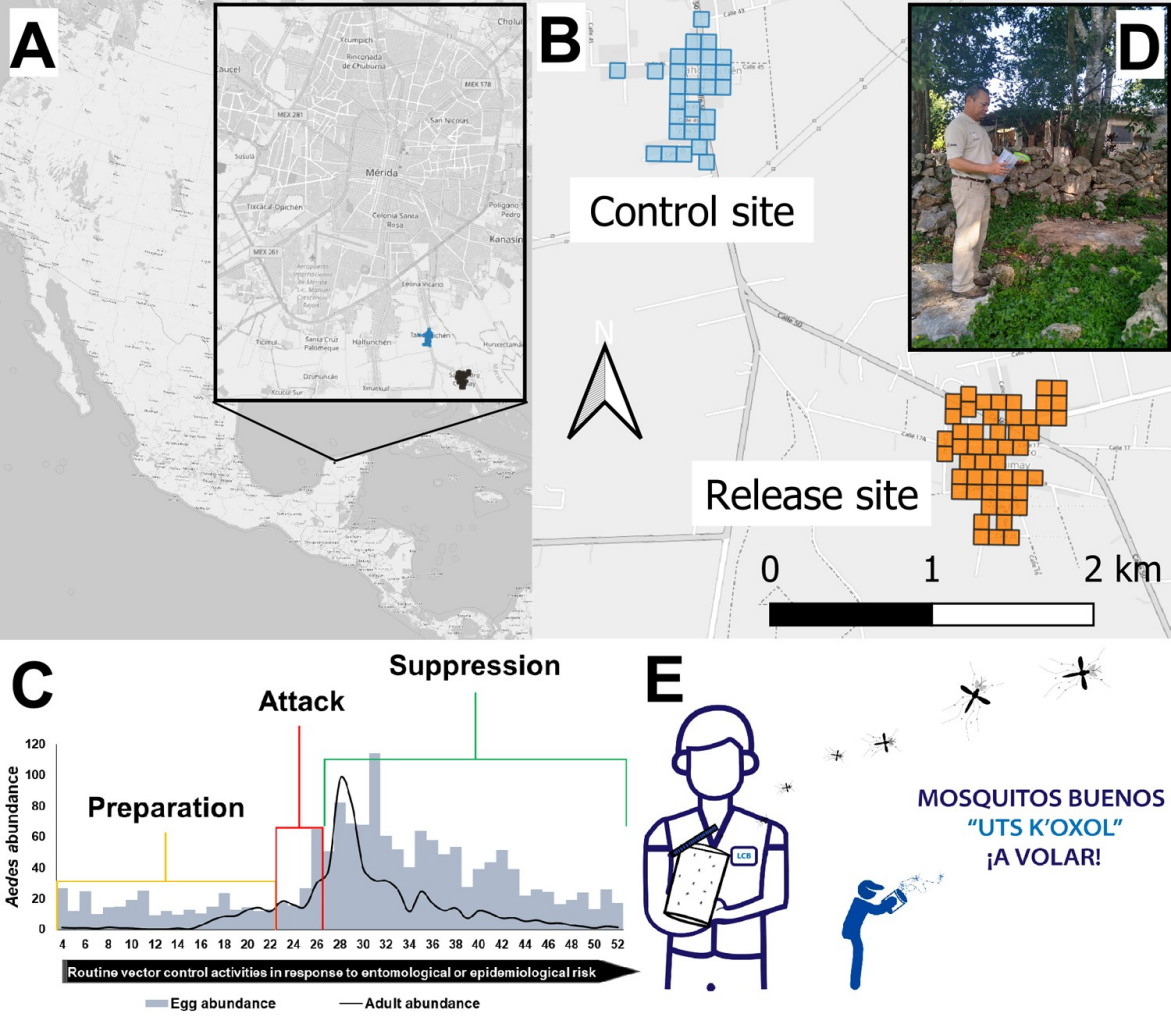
A) Field sites located in the periphery of the city of Merida, southeast of Mexico; San Pedro Chimay (release site) shown in orange and Tahdzibichén (control site) in blue; B) Distribution of one-hectare areas within localities, where sentinel areas for entomological surveillance were located and releases were performed (only at San Pedro Chimay); C) Proposed an Integrated Vector Management plan combining ‘traditional *Aedes* control’ and the release of X-ray irradiated male *Ae*. *aegypti* carrying *Wolbachia* for population suppression structured in three phases: Preparation: with community sensitization and engagement, and baseline entomological studies; Attack: initial traditional vector control; and Suppression phase: with inundative releases during peak of mosquito abundance; D) Sterile male mosquitoes released in a backyard by personnel of the MoH; E) "Uts koxol” (“good mosquitoes” in Mayan language). Maps were produced using QGIS based on public geographic data obtained from OpenStreetMap (www.openstreetmap.org).
